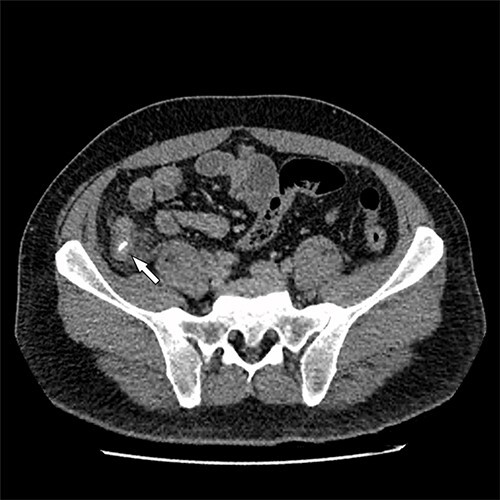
Axial CT image. Arrow indicates appendicolith present within the appendix, with minor fat stranding surrounding the appendix.
